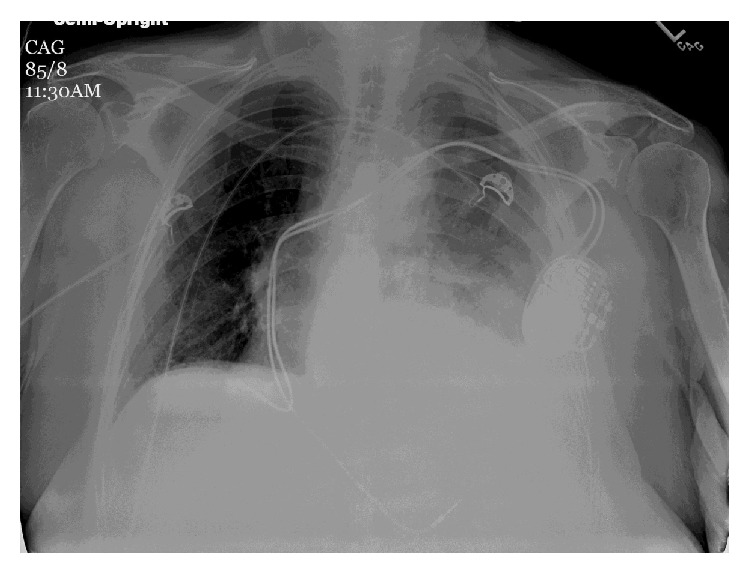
Chest X-ray AP view: opacity at left base consistent with a moderate left-sided pleural effusion; pacemaker leads appear to be present in the right ventricle.
